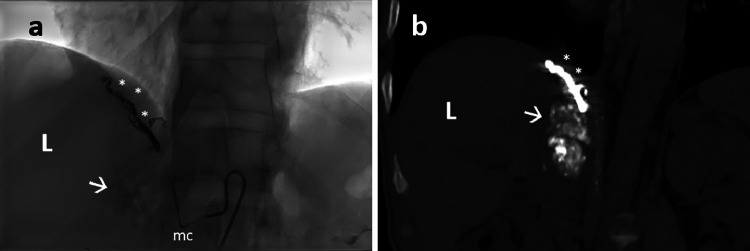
TACE performed through a diaphragmatic right artery. The distal part of the artery was coiled (asterisks) to avoid non target chemoembolization. Then, the emulsion of Lipiodol and doxorubicine was injected (arrow) until flow stasis. a: fluoroscopy image; b: coronal unenhanced CT image after treatment; L: liver; mc: microcatheter.
